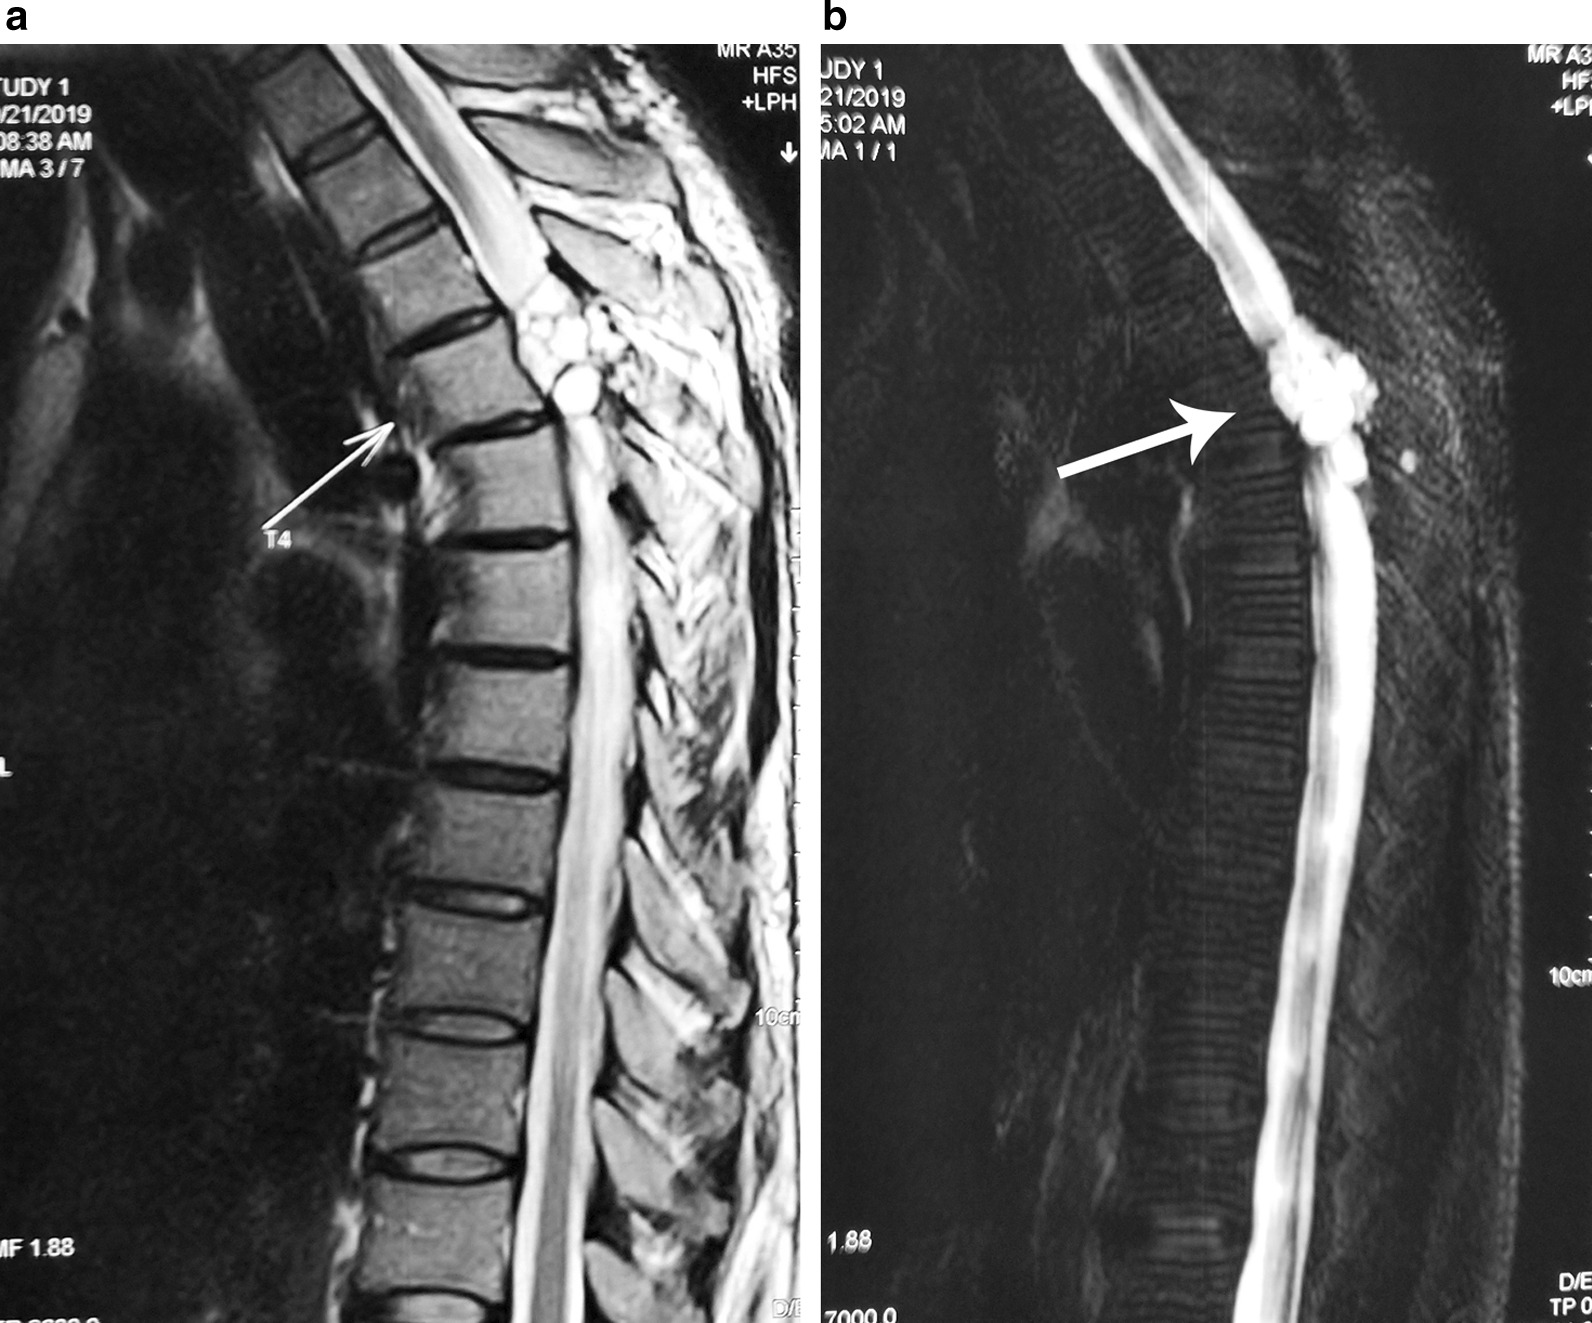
a Sagittal magnetic resonance imaging image at the level of the second thoracic vertebra of the patient, showing a lesion consisting of multiple cysts (white arrow) in the thoracic spinal cord. b Two-dimensional myelogram image showing a multiple cystic lesion (white arrow)
